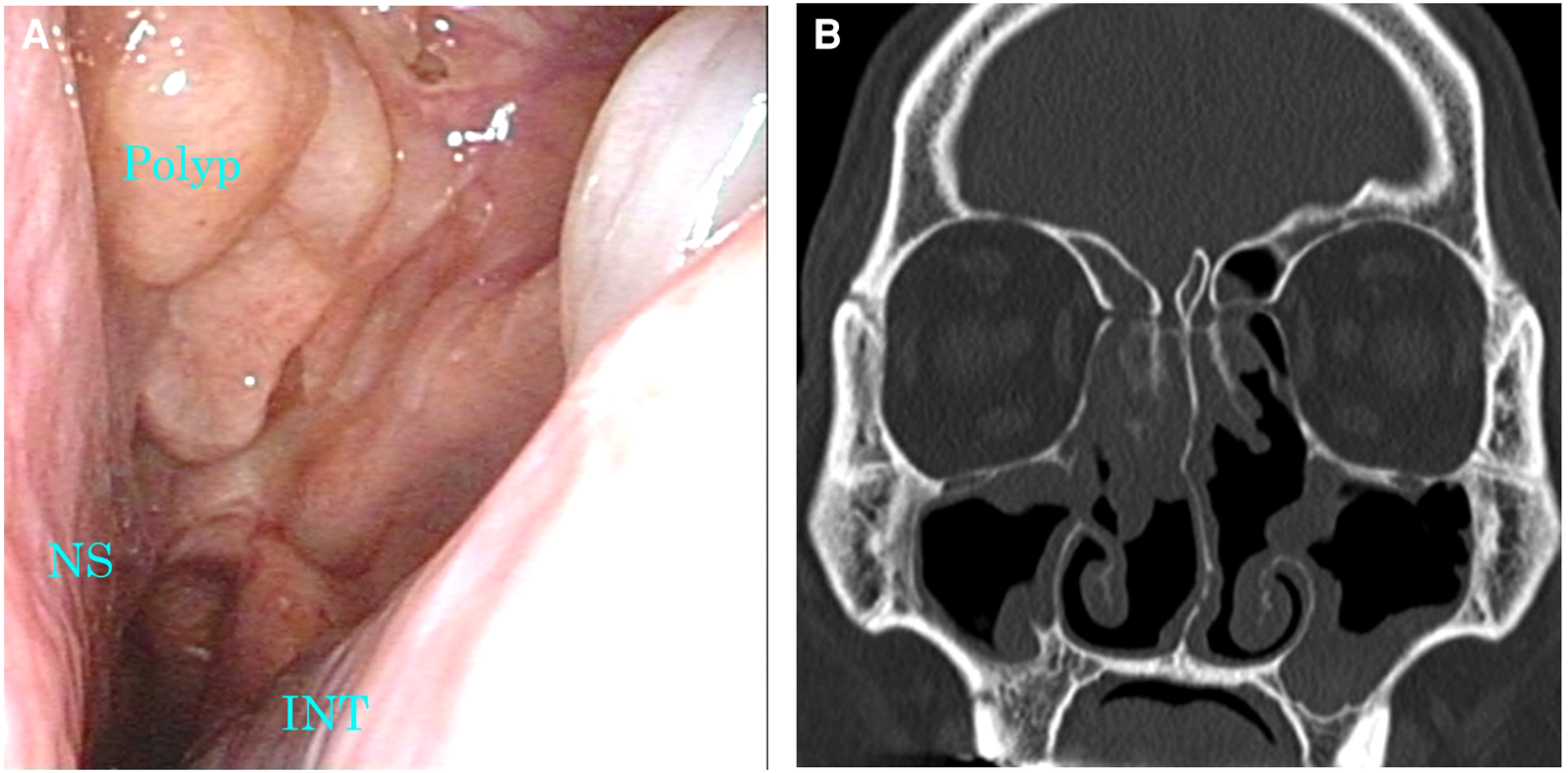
Images of nasal endoscopy and sinus CT 1 month after the diagnosis of SARS-CoV-2 infection. (A) A marked reduction of nasal polyps was confirmed. (B) Soft tissue density lesions in the sinuses were markedly reduced. CT, computed tomography; INT, inferior nasal turbinate; NS, nasal septum; SARS-CoV-2, severe acute respiratory syndrome coronavirus 2.
